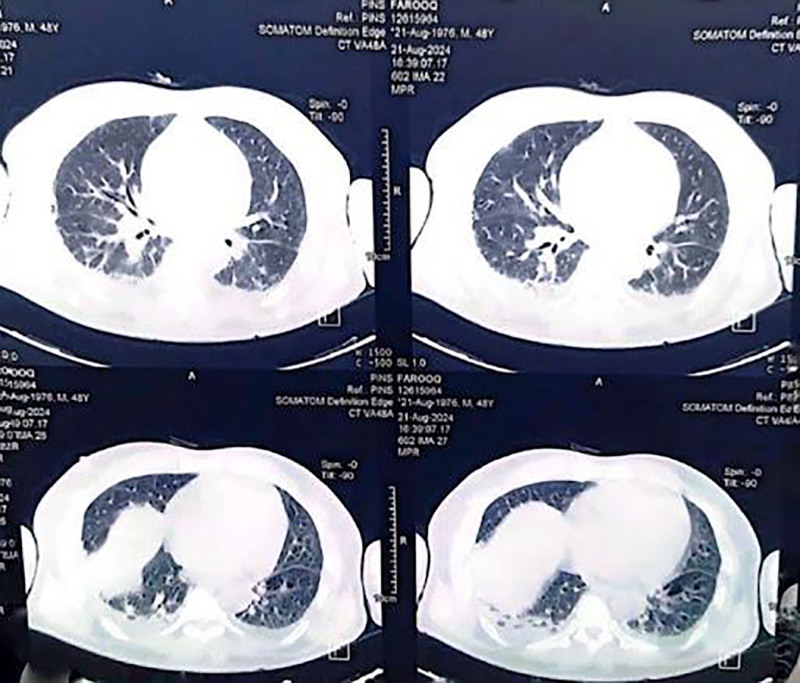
HRCT chest shows interstitial thickening and emphysematous changes in both lungs. HRCT = high-resolution computed tomography.
